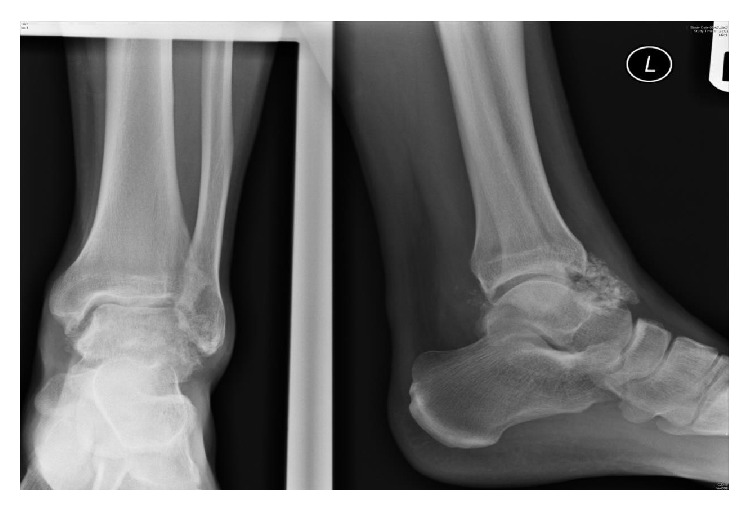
Preoperative radiographs showing a large speculated mass of calcified loose bodies.
